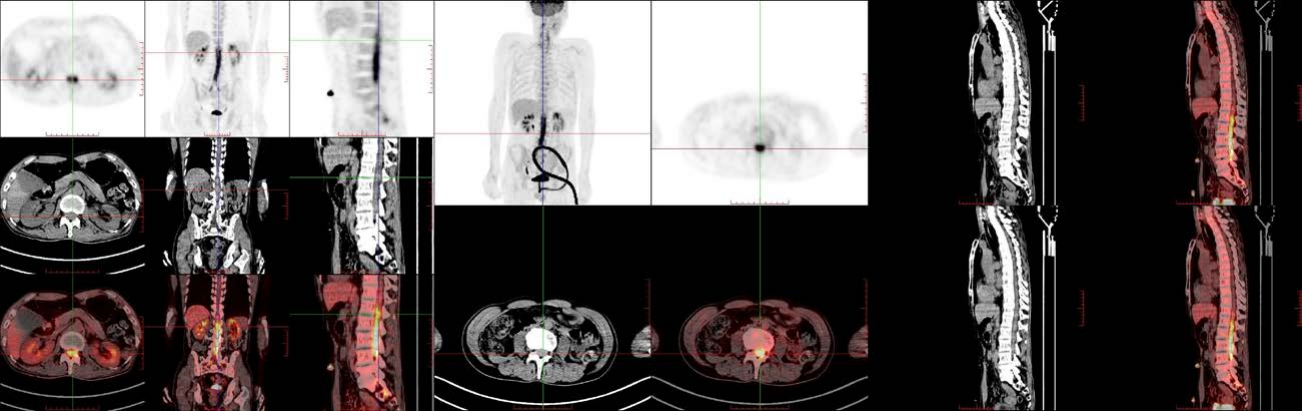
PET-CT image. The detection of hypermetabolic regions could provide indications for PCNS-LBCL and further emphasize the importance of cerebrospinal fluid analysis. PCNS-LBCL = primary central nervous system large B-cell lymphoma, PET-CT = positron emission tomography–computed tomography.
